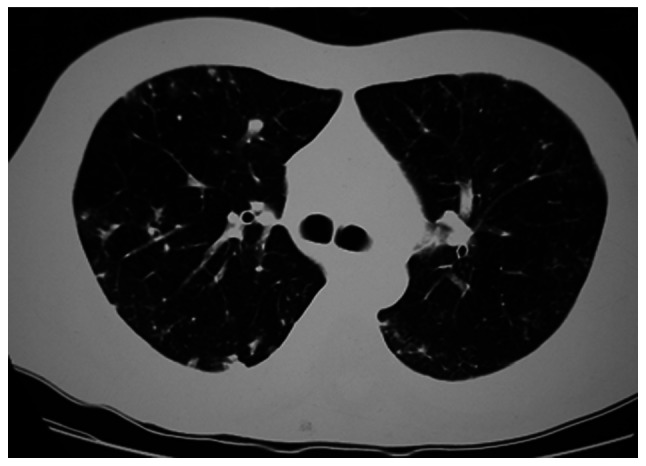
Thoracic computed tomography (CT) reveals multiple bilateral nodules of different sizes. Some of the nodules are surrounded by ground-glass opacities.
